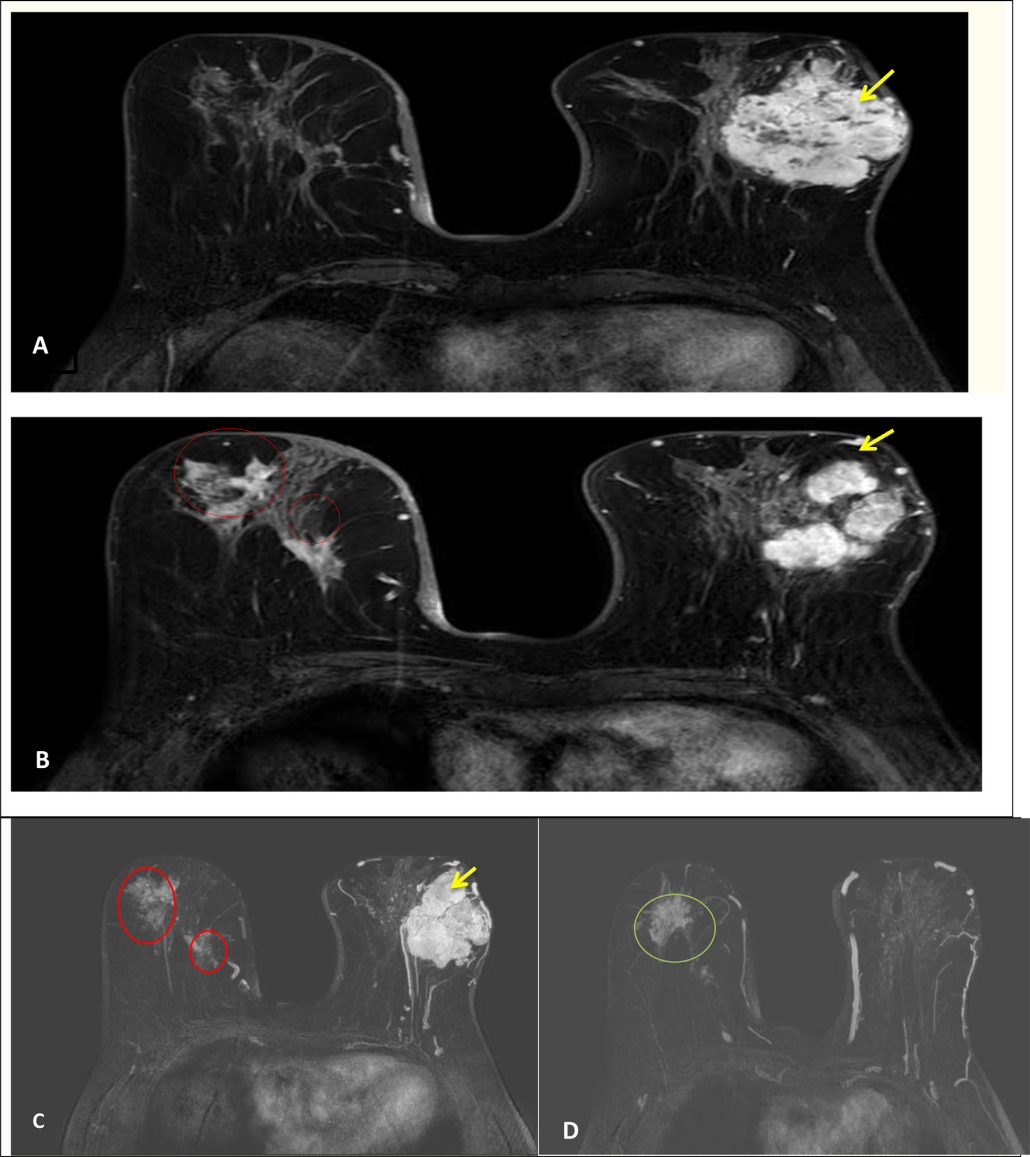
Axial postcontrast T1 FAT SAT (A, B) Axial 3D Maximal Intensity Projection (C, D) breast MRI showing in the left breast a lesion with irregular margins and heterogeneous enhancement (yellow arrow), in the right breast 2 lesions with spiculated margins (red circle), and MRI has shown multicentricity on the left upper outer quadrant (green circle).
